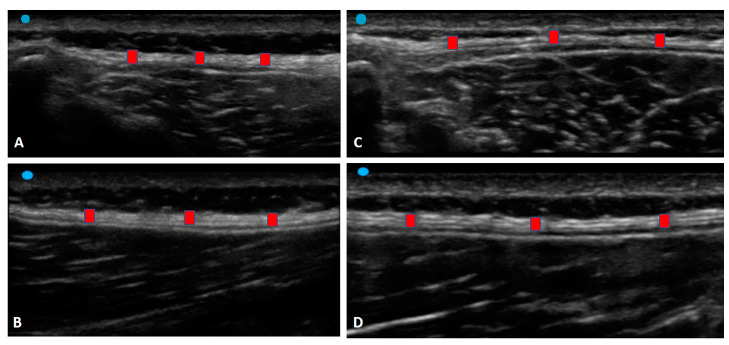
Ultrasound images of the TLF thickness: (A) Group 1: transverse axis; (B) Group 1: longitudinal axis; (C) Group 2: transverse axis; (D) Group 2: longitudinal axis. Red square: TLF.
